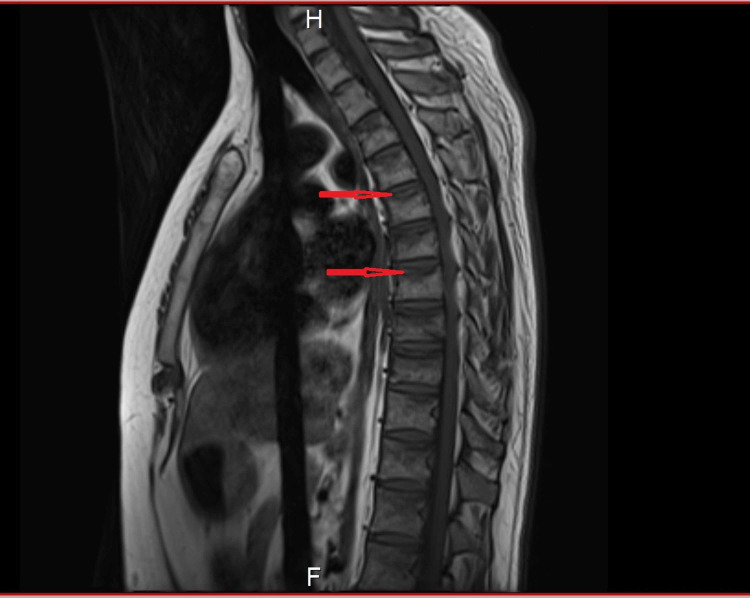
Sagittal slice of a T1-weighted MRI showing multiple vertebral compression fractures along the superior end plates (red arrows)
